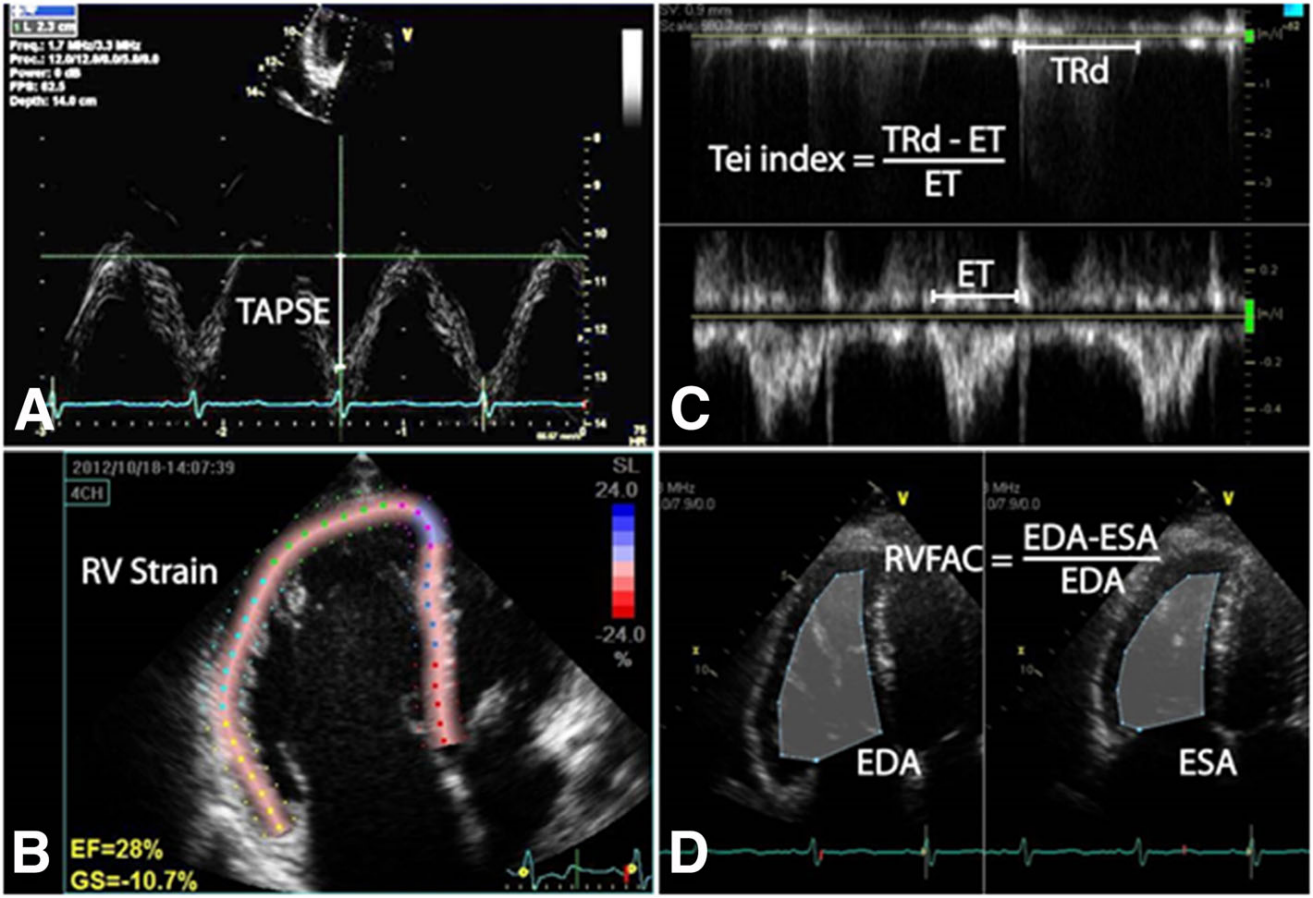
Different 2D echocardiographic methods used in this study to assess RV function. a Tricuspid Annular Plane Systolic Excursion (TAPSE) is determined from an M-mode image through the lateral tricuspid annulus by calculating the amount of longitudinal motion of the tricuspid annulus at peak systole. b RV longitudinal strain is calculated from speckle-tracking of an RV focused apical 4-chamber view. c RV Tei index is calculated as the RV isovolumic time (equal to tricuspid regurgitation duration (TRd) – ejection time (ET)) divided by the ET using the pulsed Doppler method. d RV Fractional Area Change (RV FAC) was calculated as the [(RV end-diastolic area – end-systolic area)/end-diastolic area] × 100
